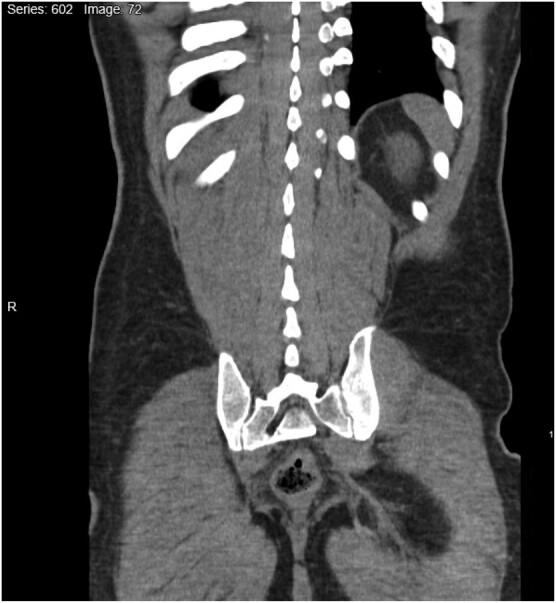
CT scan showing prostatitis with probable prostatic abscess.
